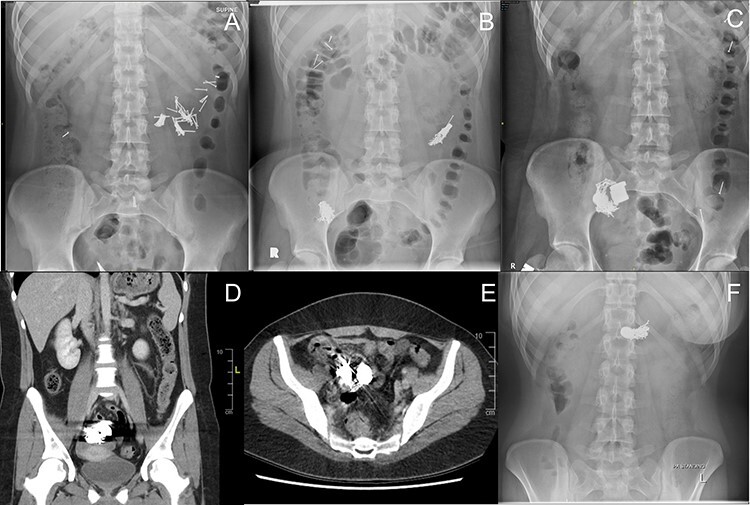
Radiographic series of sequentially ingested nails and magnets. The nails measured 2 cm long and magnets 2 cm in diameter and 0.75 cm in height. All dates are relative to initial ingestion of nails. (A) Day 1 – Nails in small bowel. (B) Day 6 – Additional ingestion of nails and a magnet. Original nails from A are clustered in right lower quadrant. (C) Day 9 – An additional four magnets have migrated to the right lower quadrant collection. (D) Day 15 – Coronal computer tomography (CT) view of nail-magnet collection in terminal ileum. (E) Day 15 – Axial CT view of nail-magnet collection in terminal ileum. (F) Day 26 – Smaller nail-magnet collection in transverse colon, 4 days after colonoscopic removal of the bulk of the collection.
